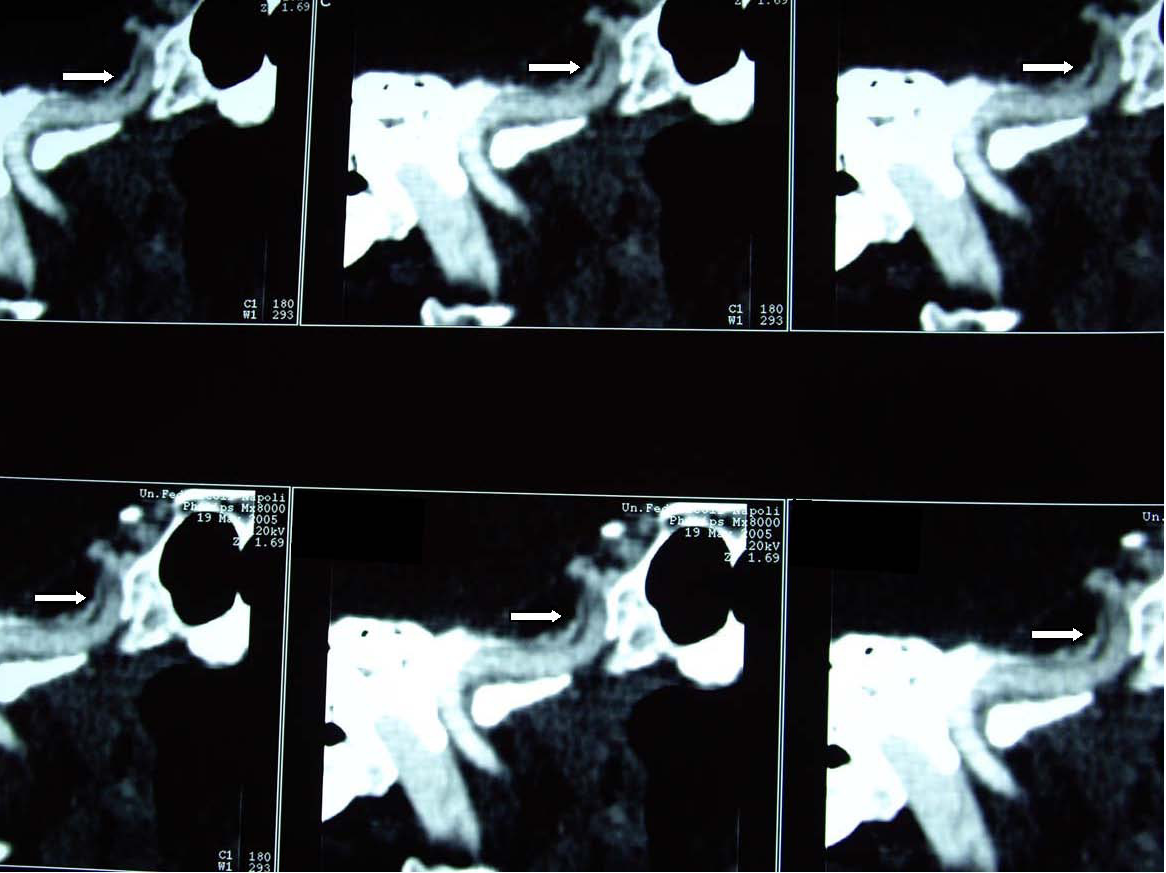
ICA computed tomography angiography. Internal carotid artery computed tomography angiography shows an irregular narrowing and an eccentric filling defect along the petrous portion of the right ICA suggestive of a dissection, as indicated by the white arrows in the sequence.
